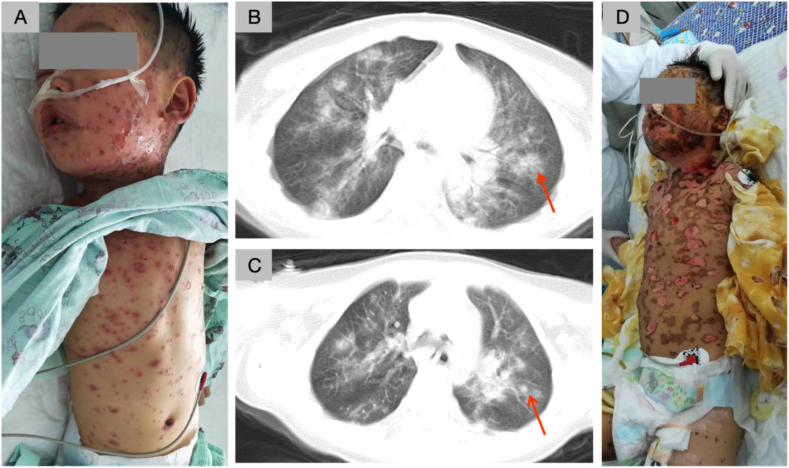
Image of patient 1 at the time of first diagnosis of TEN after admission. (A) Translucent blisters or ecchymosis-like changes appeared in the center of some rashes, especially on the head, face, trunk, and proximal limbs. (B–C) Lung computed tomography (CT; Philips Ingenuity microplate CT) was performed after endotracheal intubation, and multiple patchy high-density shadows, bronchial thickening, and local lung consolidation were observed in both lungs. Due to the presence of jitter in the CT radiographs of the child, the image appears fuzzy, and the annotated image contains artifacts. The red arrow indicates local lung consolidation. (D) After treatment in the hospital, the skin and mucous membrane damage worsened. (For interpretation of the references to colour in this figure legend, the reader is referred to the Web version of this article.)
